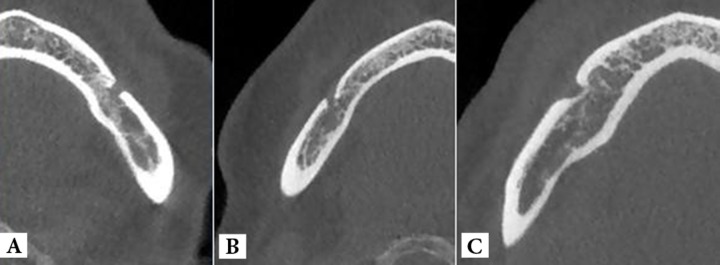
Mandibular canal emerging to mental foramen on surface of mandibular body in axial CBCT images. There are three emergence types; *A*) straight, *B*) anterior and *C*) posterior
